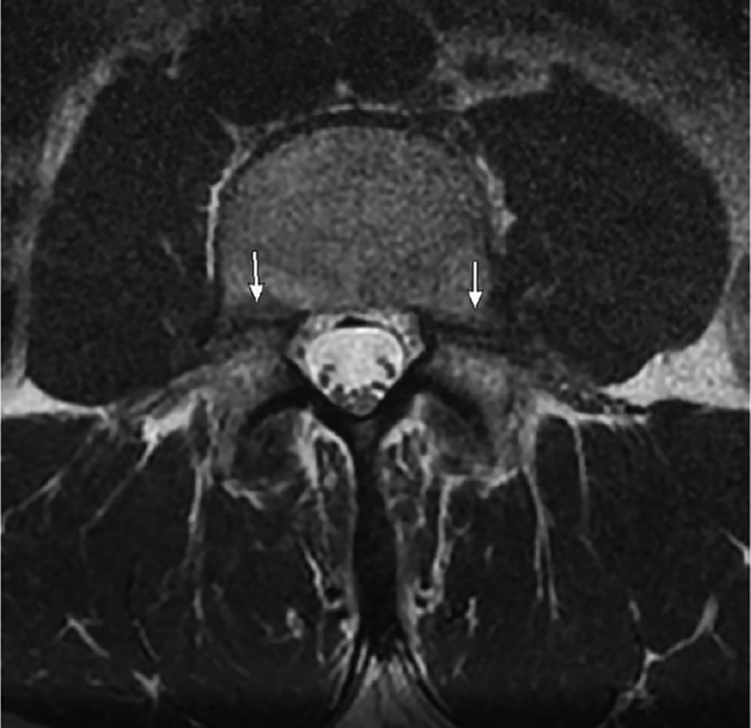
Axial T2-weighted MRI of L4 depicting bilateral pedicle fractures (straight arrow). No appreciable marrow edema.
